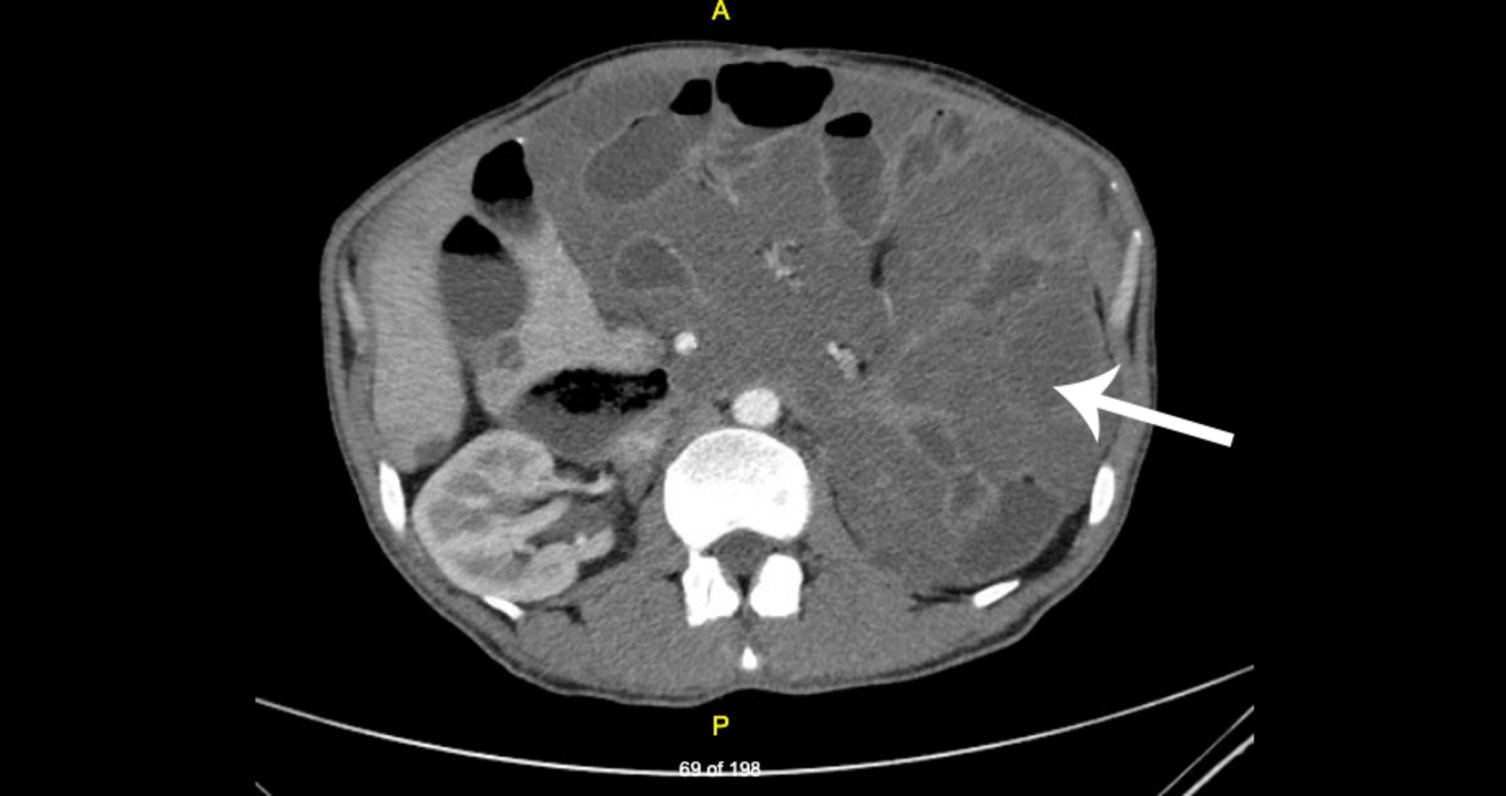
CT scan of abdomen and pelvis with contrast Diffuse pseudomyxoma peritonei in the peritoneal cavity (solid arrow) CT: computed tomography
